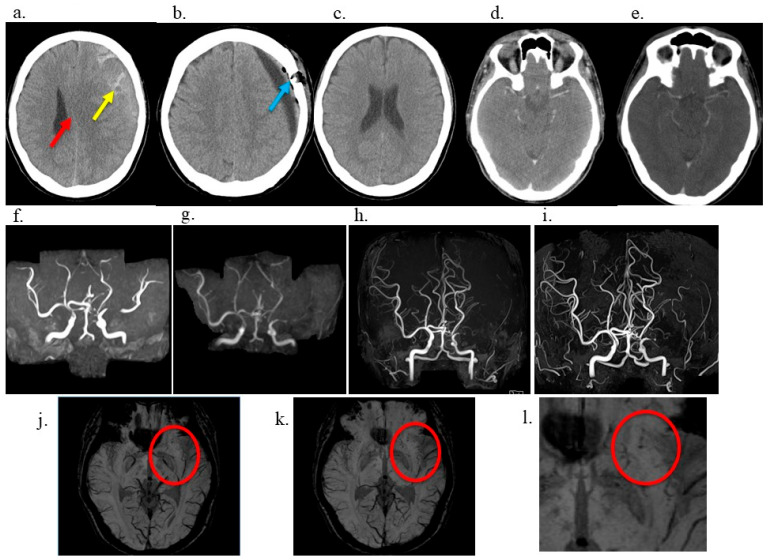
Head computed tomography (CT) or magnetic resonance imaging showing chronic subdural hematoma (CSDH) or progression of left middle cerebral artery (MCA) stenosis. (a) Preoperative head CT showing a left-sided CSDH (yellow arrow) with midline shift (red arrow). (b) Postoperative day 1 CT demonstrating resolution of the hematoma with adequate drainage (drain tube and burr hole: bule arrow) and improved midline shift. (c) CT obtained 1 month following surgery showing complete resolution of the hematoma and normalized brain contour. (d) Reference contrast-enhanced CT at 37 years old before CSDH showing symmetrical bilateral middle cerebral arteries (MCAs) without stenosis. (e) Contrast-enhanced CT at 41 years old, 1 year after CSDH, demonstrating no stenosis of the left MCA. (f) MRA at 43 years old, 3 years after CSDH, demonstrating mild stenosis of the left MCA. (g) MRA at 46 years old, 6 years after CSDH, showing significant progression of left MCA stenosis. (h) MRA at 47 years old, 7 years after CSDH, revealing non-visualization of the left MCA. (i) MRA at 50 years old, 10 years after CSDH, confirming continued non-visualization of the left MCA. (j,k) SWI demonstrating Moyamoya-like collateral vessels (red elliptical) in the left cerebral hemisphere suggestive of Moyamoya-like vascular networks. (l) Magnified SWI view corresponding to panel (k), demonstrating abnormal fine collateral vessels (red elliptical) in the left basal ganglia. These findings are characteristic of Moyamoya-like vasculopathy and were not observed on the contralateral side.
